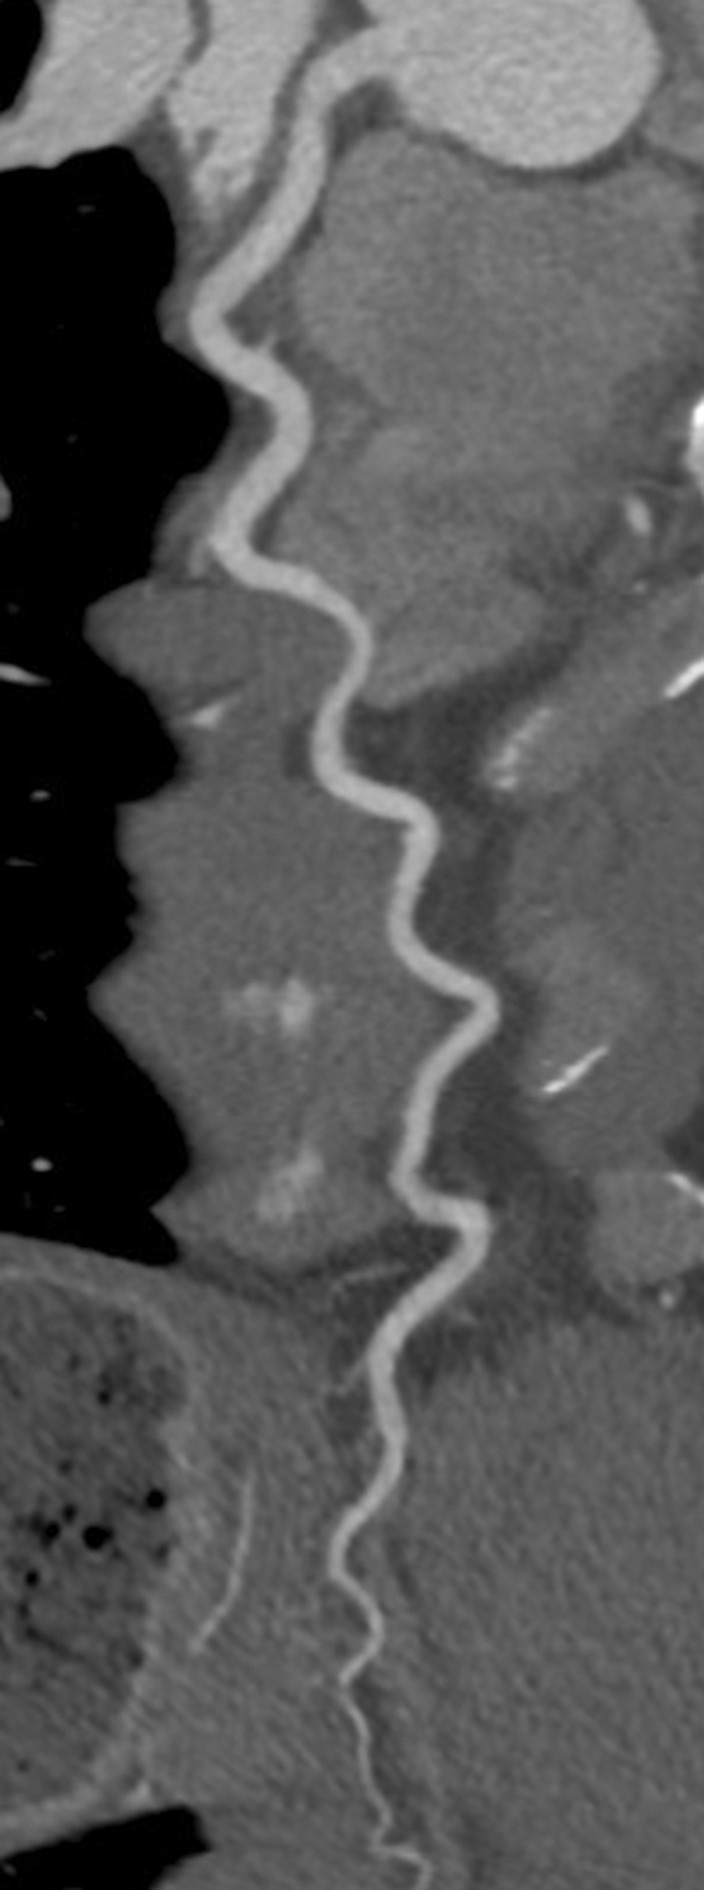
Multiplanar curved reformat of CT coronary angiogram Figure showing the large calibre left anterior descending artery (LAD) with a tortuous course and continuing as the posterior descending artery (PDA) distally. No significant coronary artery disease was identified.
